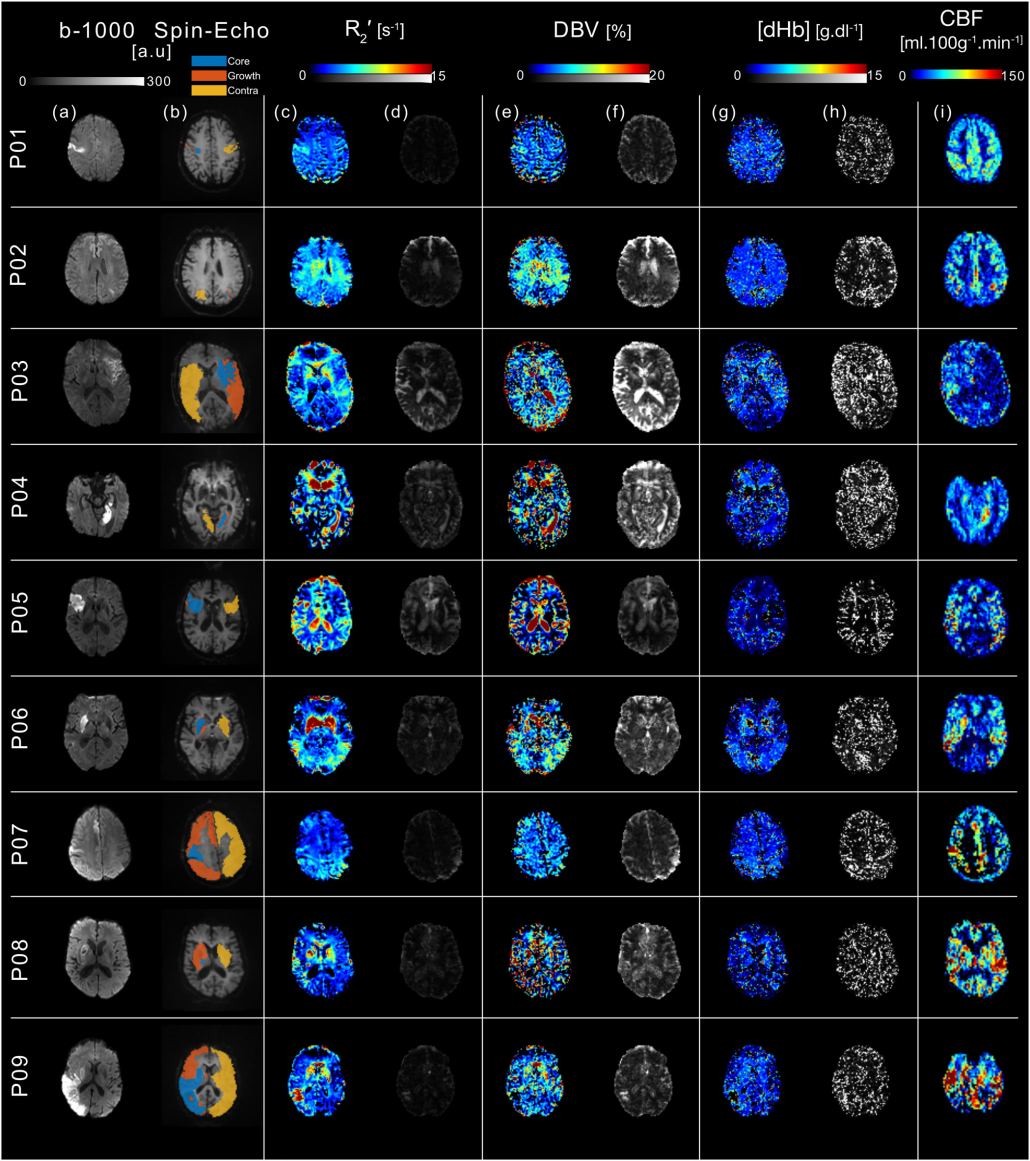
Data acquired during initial MRI scanning for all patients included in the study (*n* = 9). (a) DWI (*b* = 1,000 s/mm^2^), (b) asymmetric spin‐echo (*τ* = 0 ms), (c–h) sqBOLD oxygenation and (i) ASL blood flow maps are presented for a single axial slice in each stroke patient. All images are presented in their native acquisition space. Core (blue), growth (orange), and contralateral (yellow) tissue outcome ROIs are displayed on the spin‐echo image of the sqBOLD acquisition (b). Streamlined‐qBOLD parameter maps (*R*
